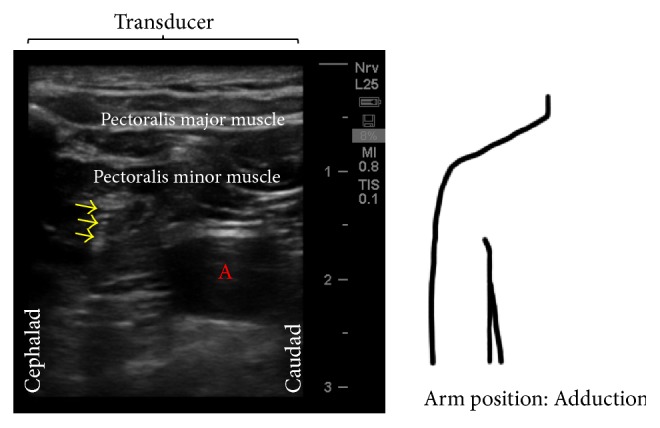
Infraclavicular neurovascular sonoanatomy with arm position adducted. The ultrasound image represents a transverse view with the top of the image displaying the ultrasound probe position. Arrows: needle shaft, A = axillary artery.
